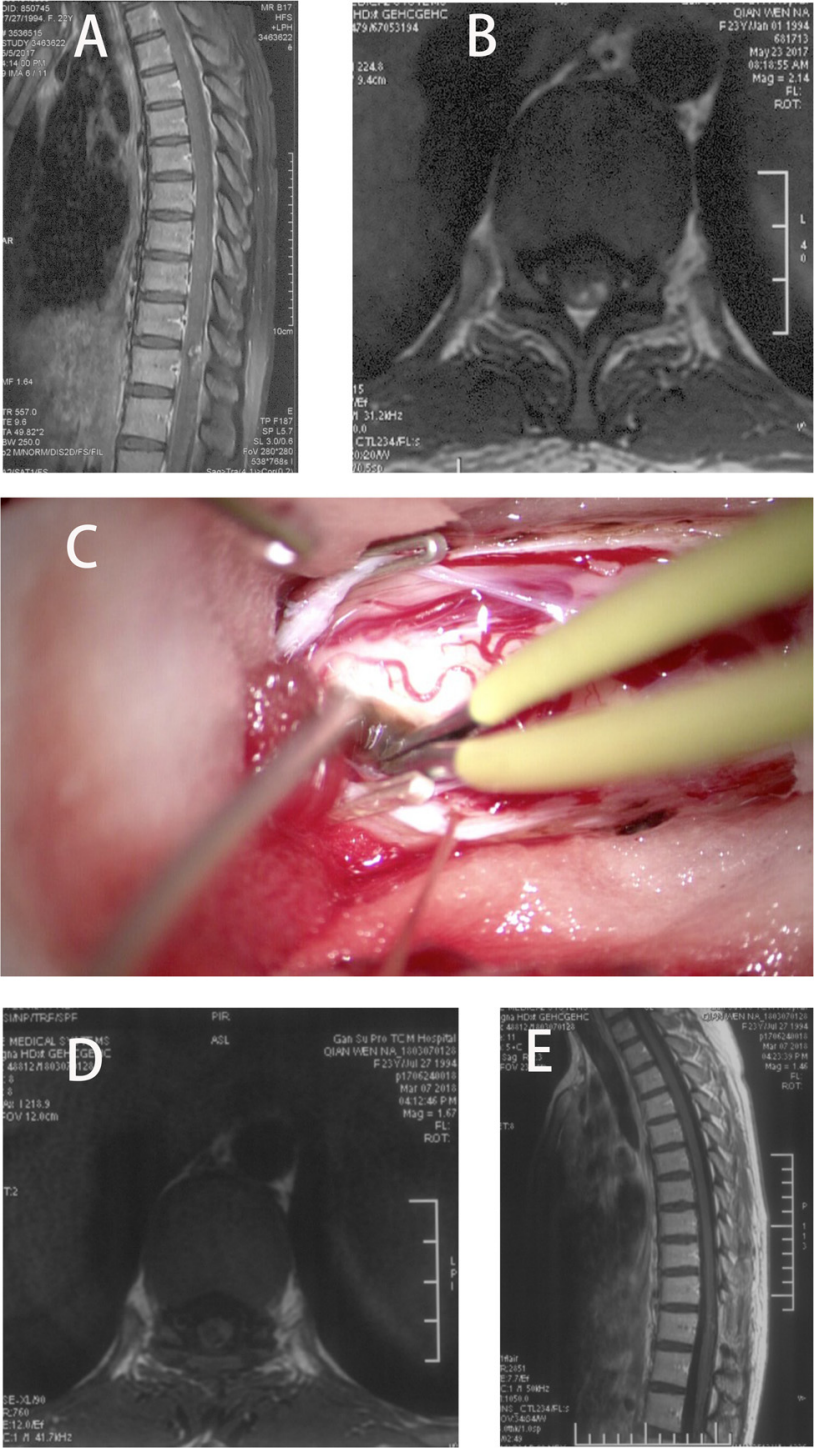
Preoperative MRI (a) and (b), and intraoperative findings (c) showed an SCM at T10–T12 level. Postoperative MRI (d) and (e).
